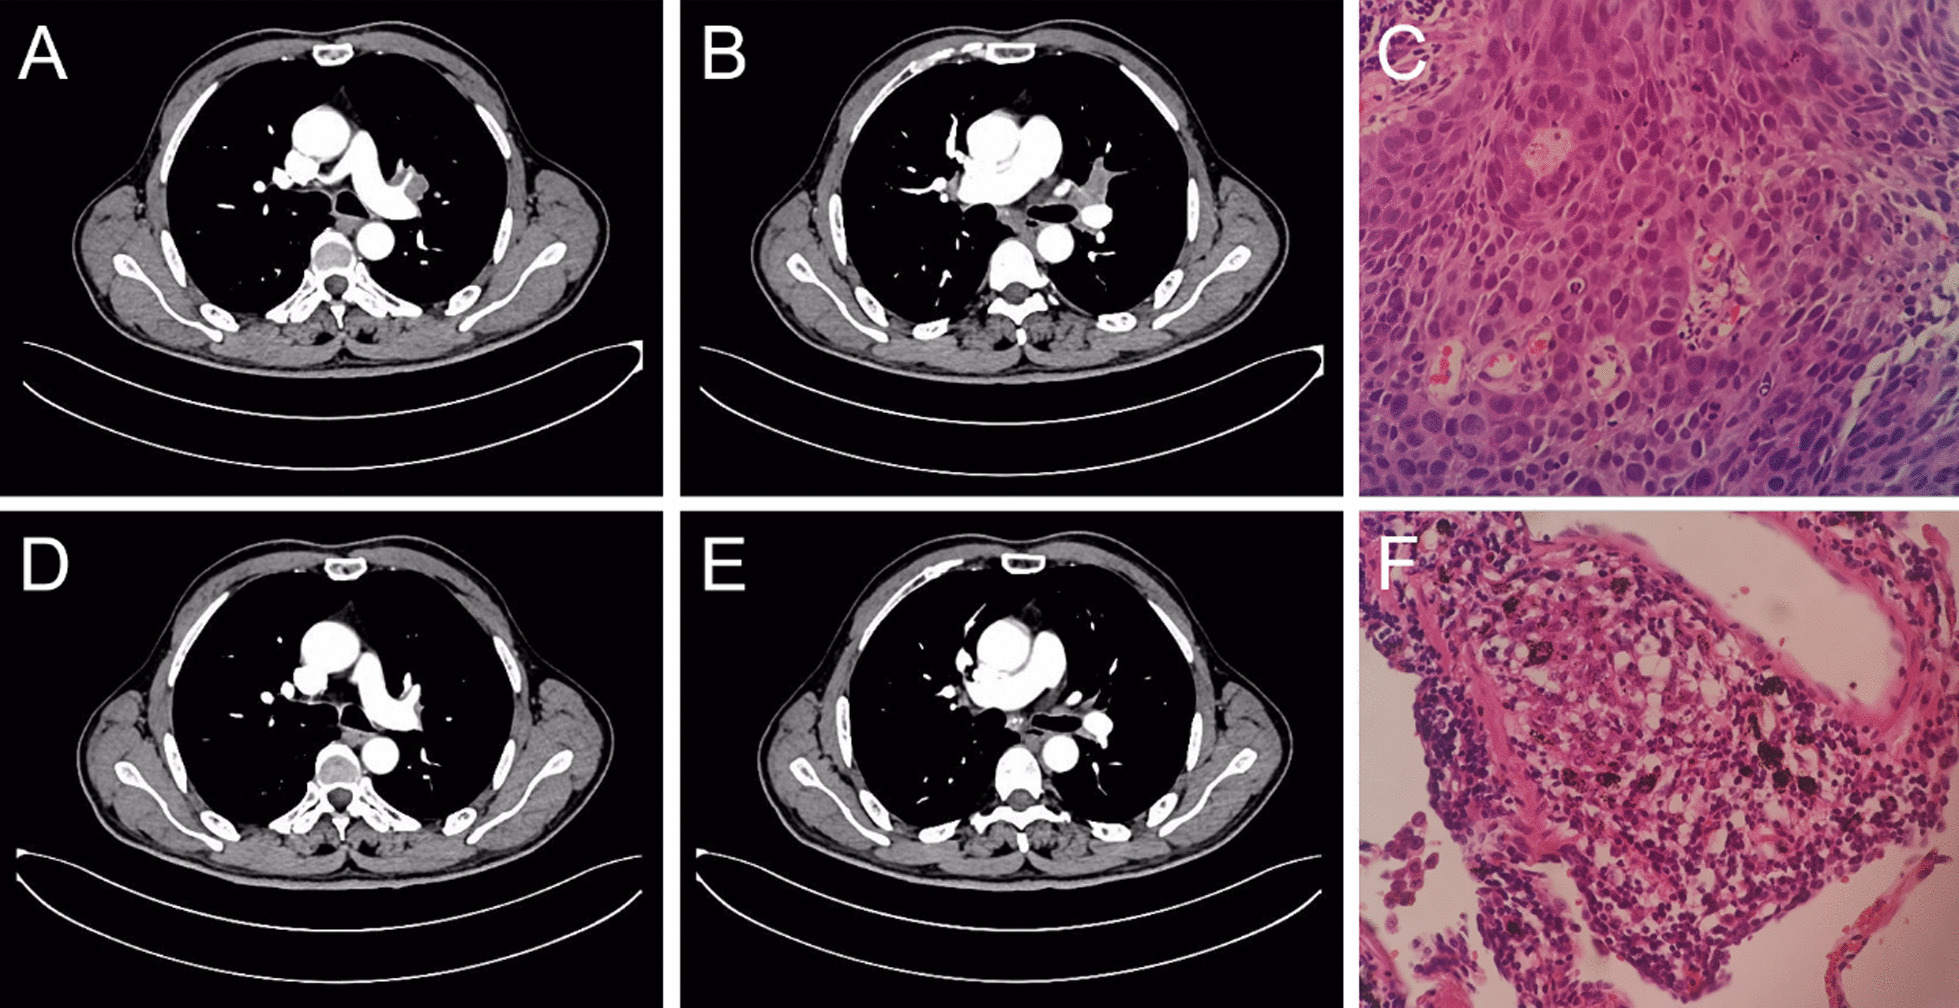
A and B are CT images before the treatments of neoadjuvant chemotherapy plus toripalimab. C is pathological image before the treatments. D and E are CT images after 3 cycles’ treatments of neoadjuvant chemotherapy plus toripalimab. F is pathological image after the treatments of neoadjuvant chemotherapy plus toripalimab followed by VATS lobectomy
